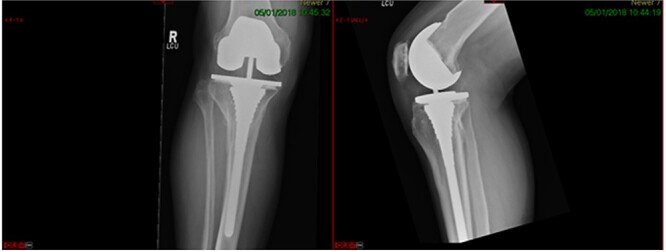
7-year post-op radiograph.
